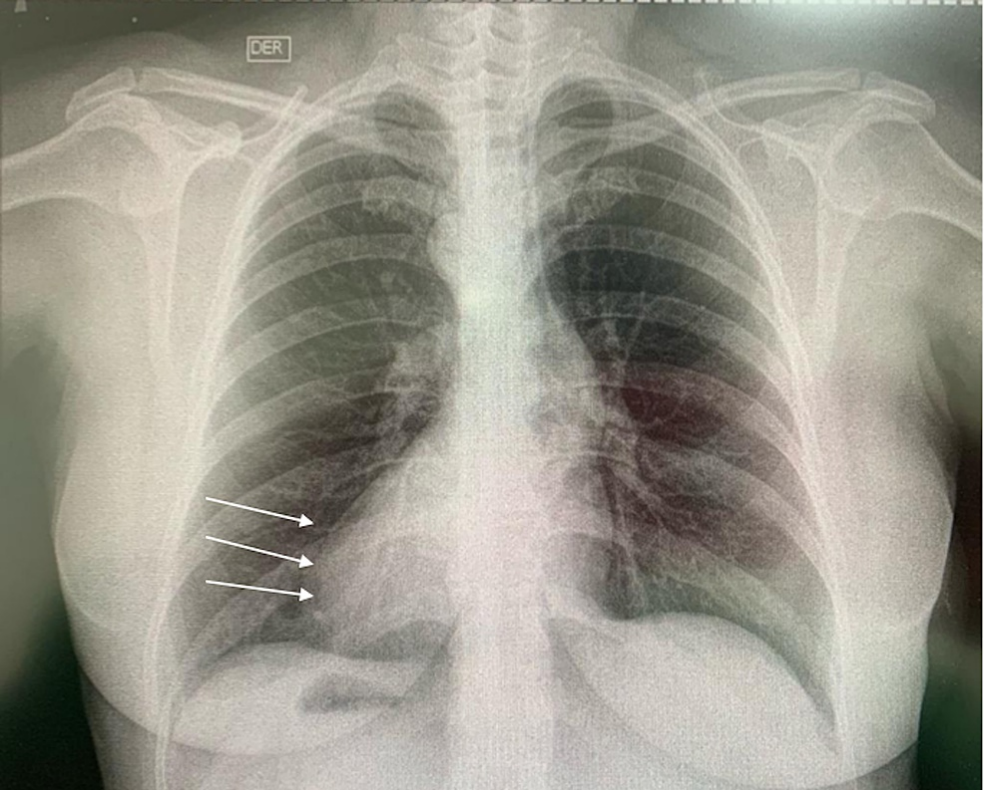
Chest radiography showing dextrocardia (white arrows)
